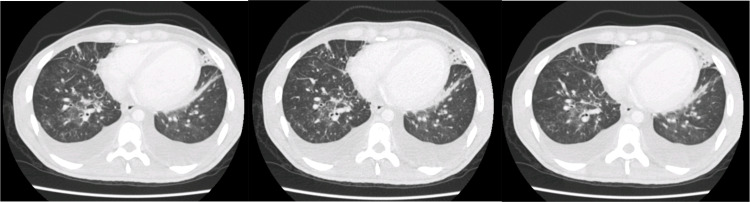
Thorax CT shows bilateral pleural effusion and nodular areas, with a ground glass pattern, associated with septal interlobular thickening CT, computed tomography
